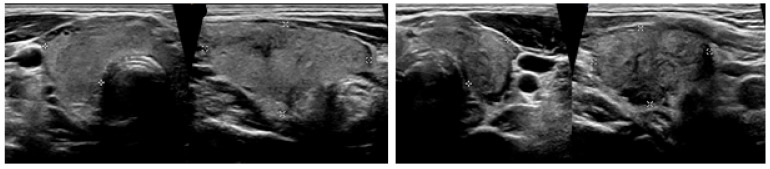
Ultrasound images at the time of the diagnosis of SAT. The total volume of the thyroid gland was about 18 mL (right lobe 10.1 mL and left lobe 7.2 mL) with heterogeneous echotexture and hypoechoic areas with ill-defined margins. SAT, subacute thyroiditis.
